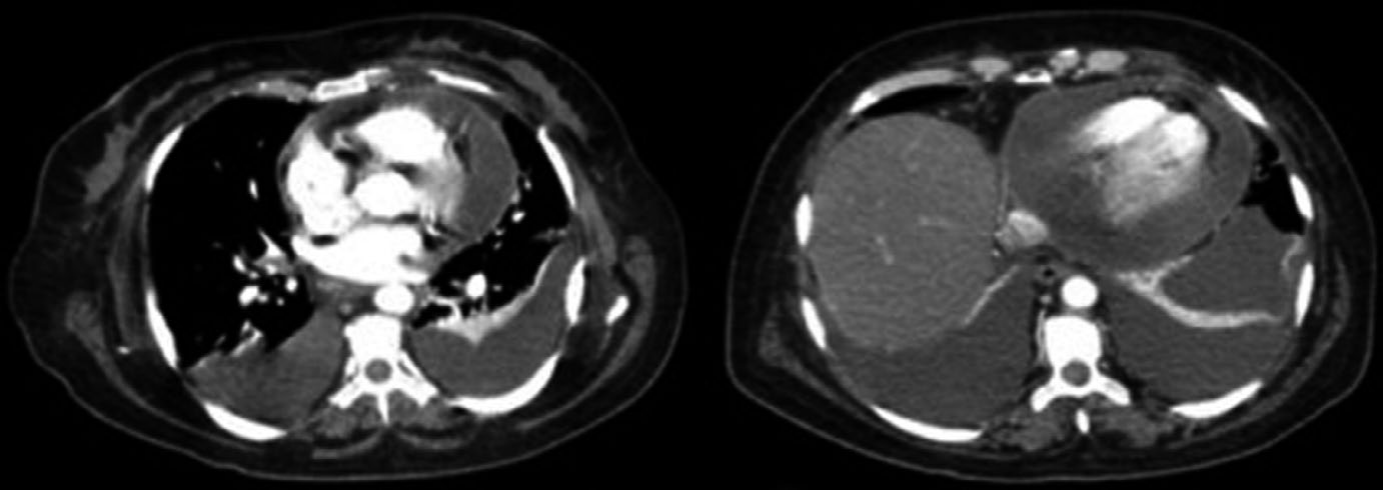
CT scan showing bilateral mediastinal lymphadenopathies and pericardial and bilateral pleural effusion
